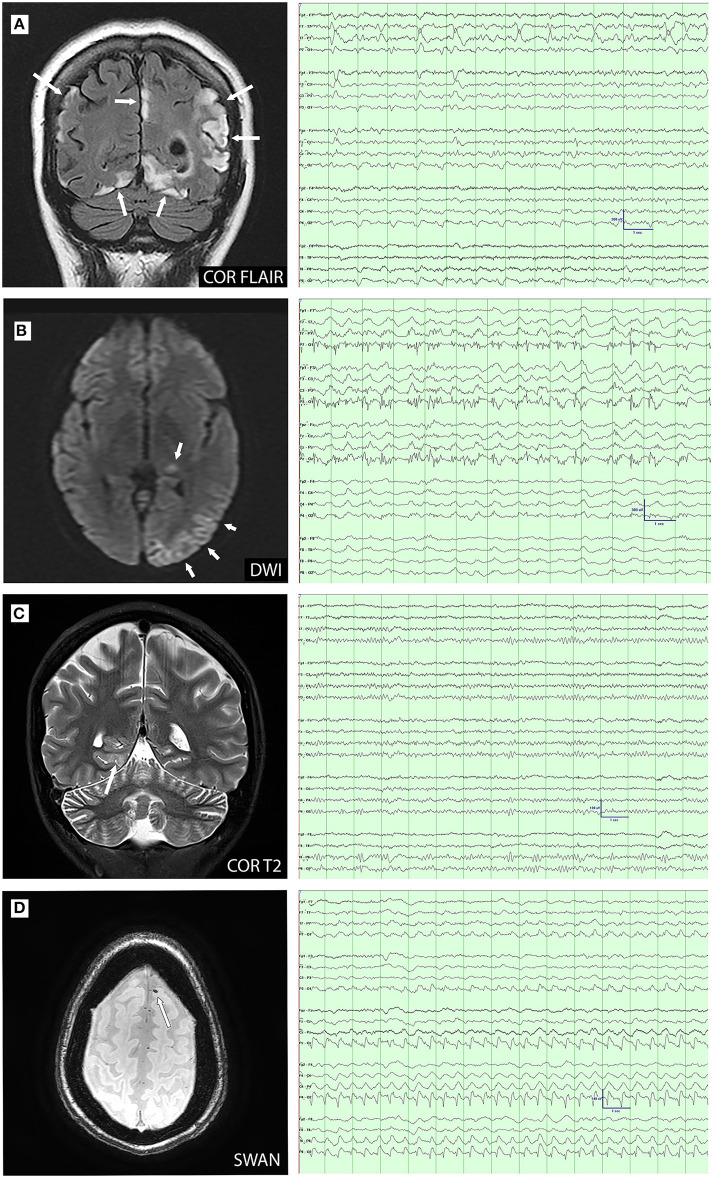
MRI-electroencephalogram (EEG) concordance (A–C) and discordance (D) comparisons. (A): Coronal FLAIR image demonstrates cortical and subcortical hyperintensity in the parietal and occipital lobes. EEG demonstrates left temporal lateral periodic discharges and bioccipital spikes; (B): Diffusion-weighted imaging (DWI) demonstrates restriction in the left pulvinar and temporo-occipital cortex. EEG shows ongoing electrographic seizure activity over the left posterior temporal and occipital; (C): Coronal T2 image demonstrates cortical hyperintensity in the right medial occipitotemporal gyrus. EEG shows right posterior temporal slowing with increased right posterior region amplitudes; and (D): Axial susceptibility-weighted angiography (SWAN) image demonstrates susceptibility artifact consistent with microhemorrhage in the left superior frontal gyrus. EEG demonstrates right posterior lateralized periodic discharges.
